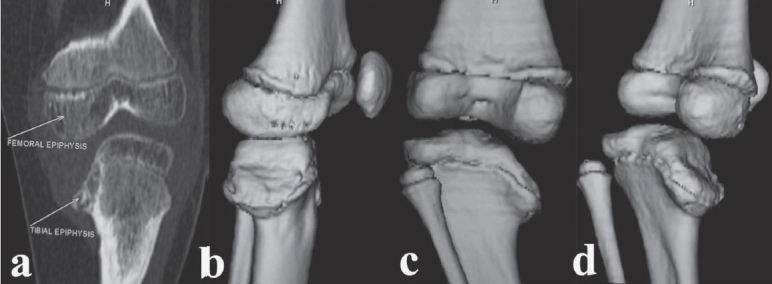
Preoperative CT scan (a) (coronal reformatted image) showing the extent of depression of the medial tibial plateau. Preoperative CT scan (b,c,d) (3D reconstruction) in various rotations demonstrating the posteromedial tibial plateau depression
